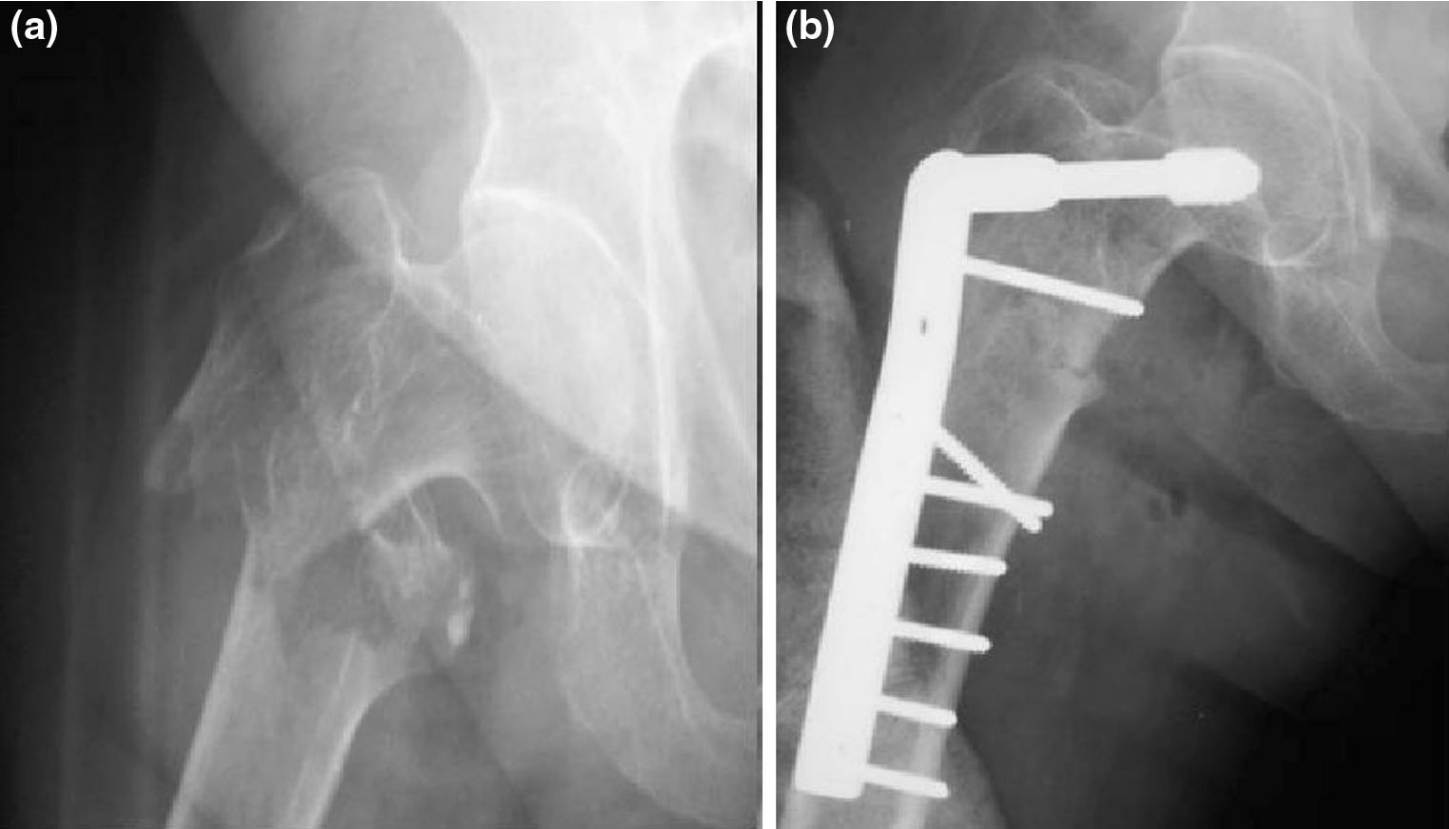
a Anteroposterior radiograph of the *right* hip of a 76-year-old female who fell in the street, revealing an 31-A3 peritrochanteric fracture. b Post op radiographs after open reduction and extramedullary fixation with a DCS
